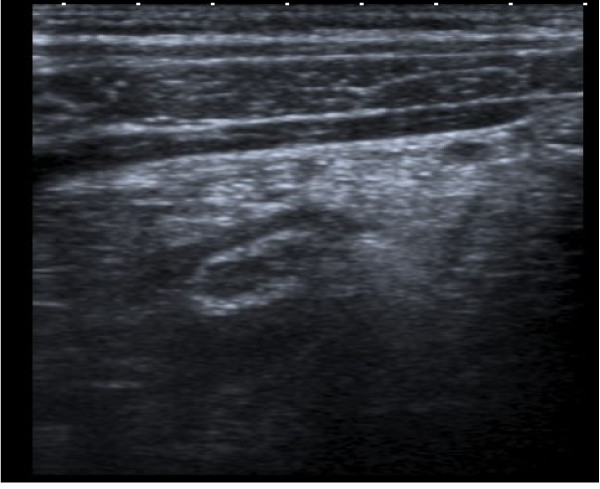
Ultrasound showing an inflamed tubular structure.
